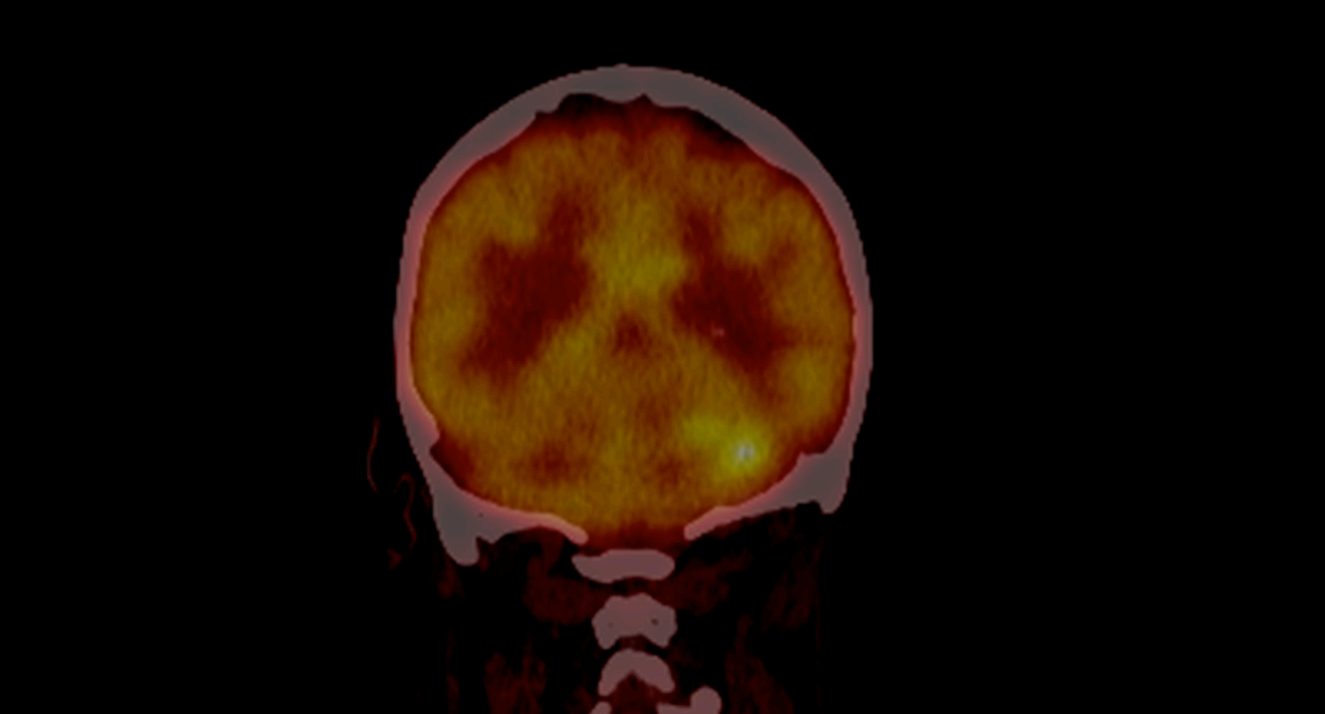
PET CT of patient 1 before treatment showing increased uptake in the cerebellum
